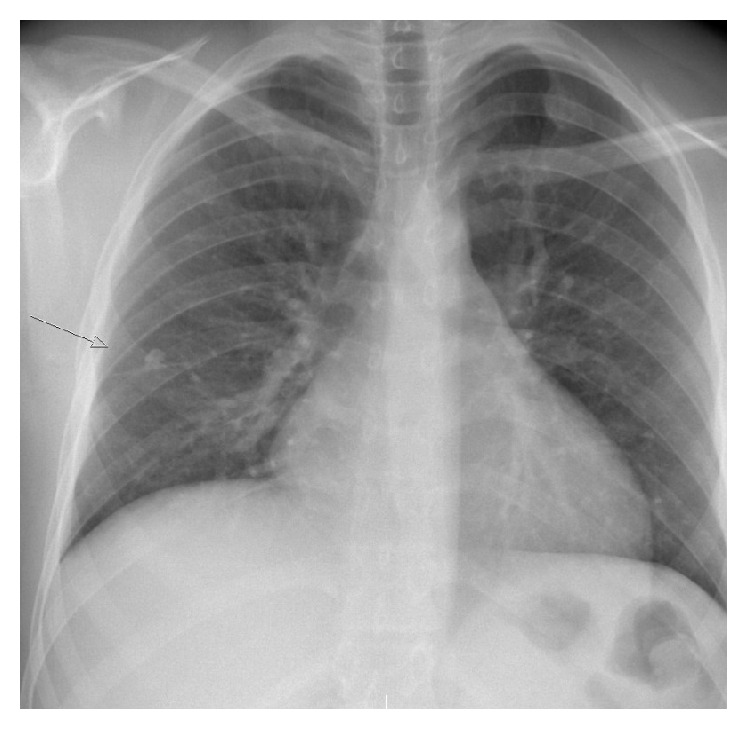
Chest X-ray showing a lesion in the right lung.
